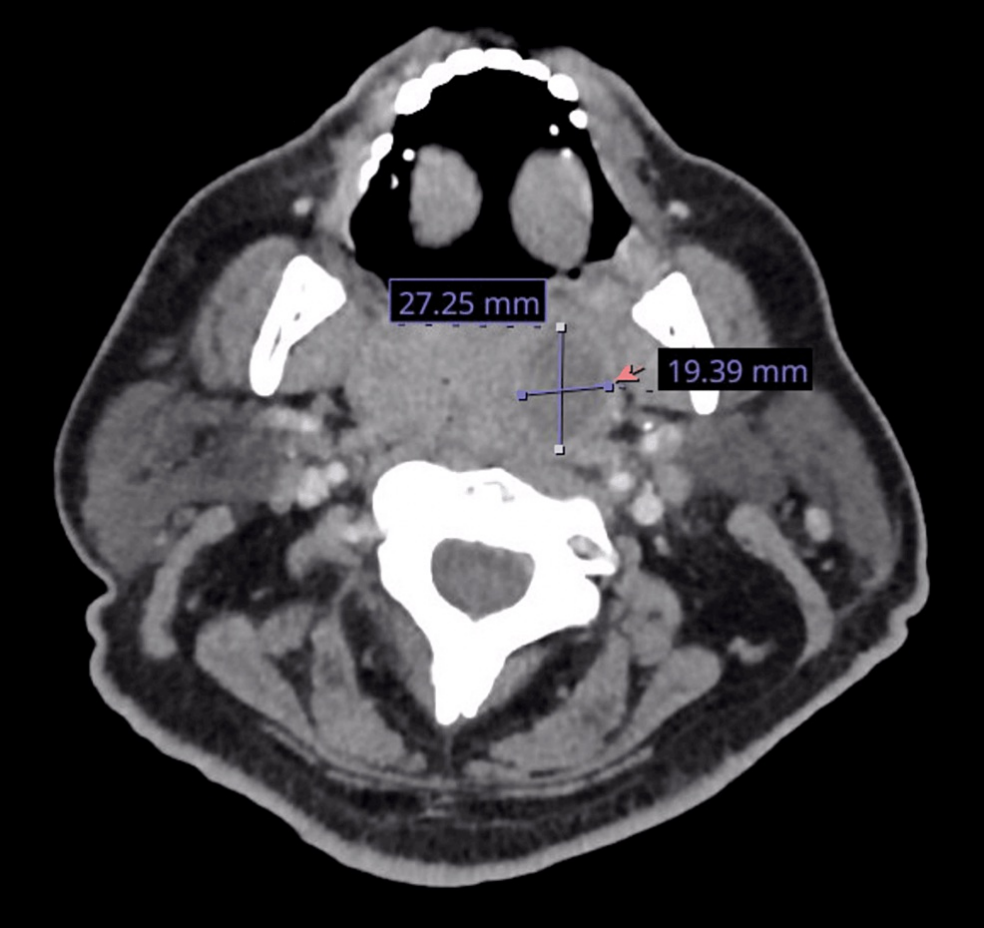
Soft tissue neck computed tomography demonstrating a large peritonsillar abscess measuring 27.25 mm x 19.39 mm (red arrow)
